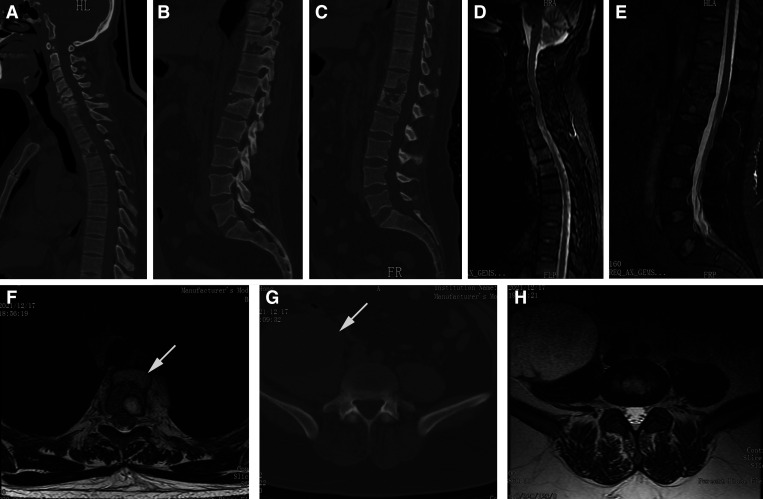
Preoperative CT and MRI scans. (A–C) Sagittal CT images indicate bony destruction in the C5, C6, T3, T10, T12, and L1 vertebrae. (D and E) Preoperative sagittal MRI indicates high signal intensity in multiple vertebrae and a fusiform paraspinal abscess in front of the T1–T4 vertebrae. (F) Axial MRI scan at the T4 vertebra level indicate a paravertebral abscess (white arrow). (G and H) Axial CT and MRI scans at the L4/5 intervertebral level indicate a massive psoas abscess (white arrow). CT = computed tomography; MRI = magnetic resonance imaging.
